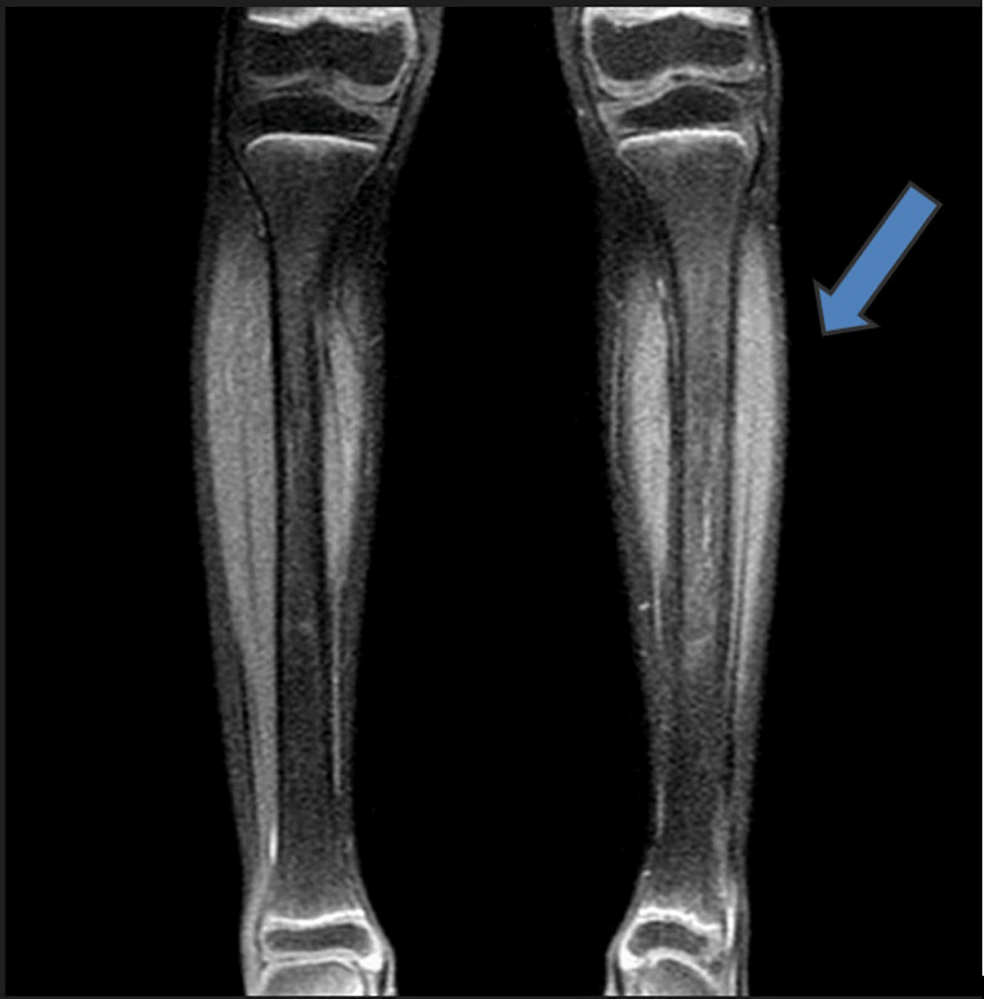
MRI revealed a patchy high stir signal in the mid-diaphysis of the left leg (tibia)
